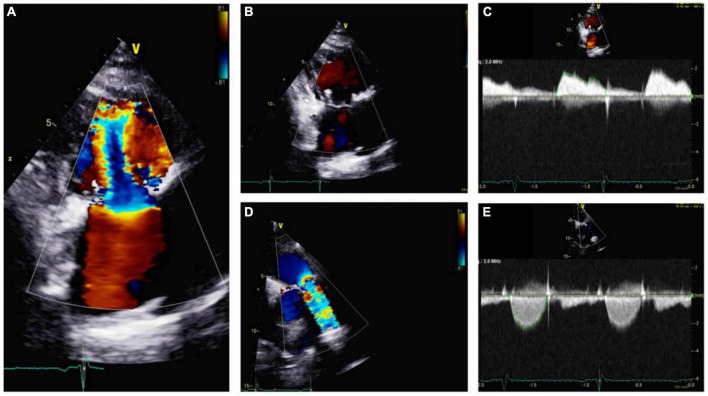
Echocardiography monitoring in carcinoid heart disease (CnHD): First follow up echo. Tricuspid valve in parasternal long axis showing mild tricuspid stenosis (A) and a lack of tricuspid regurgitation (B); continuous wave color Doppler shown (C). Pulmonic valve is shown in color Doppler (D) and continuous wave color Doppler, where there is evidence of increased pulmonary valve velocity (E).
